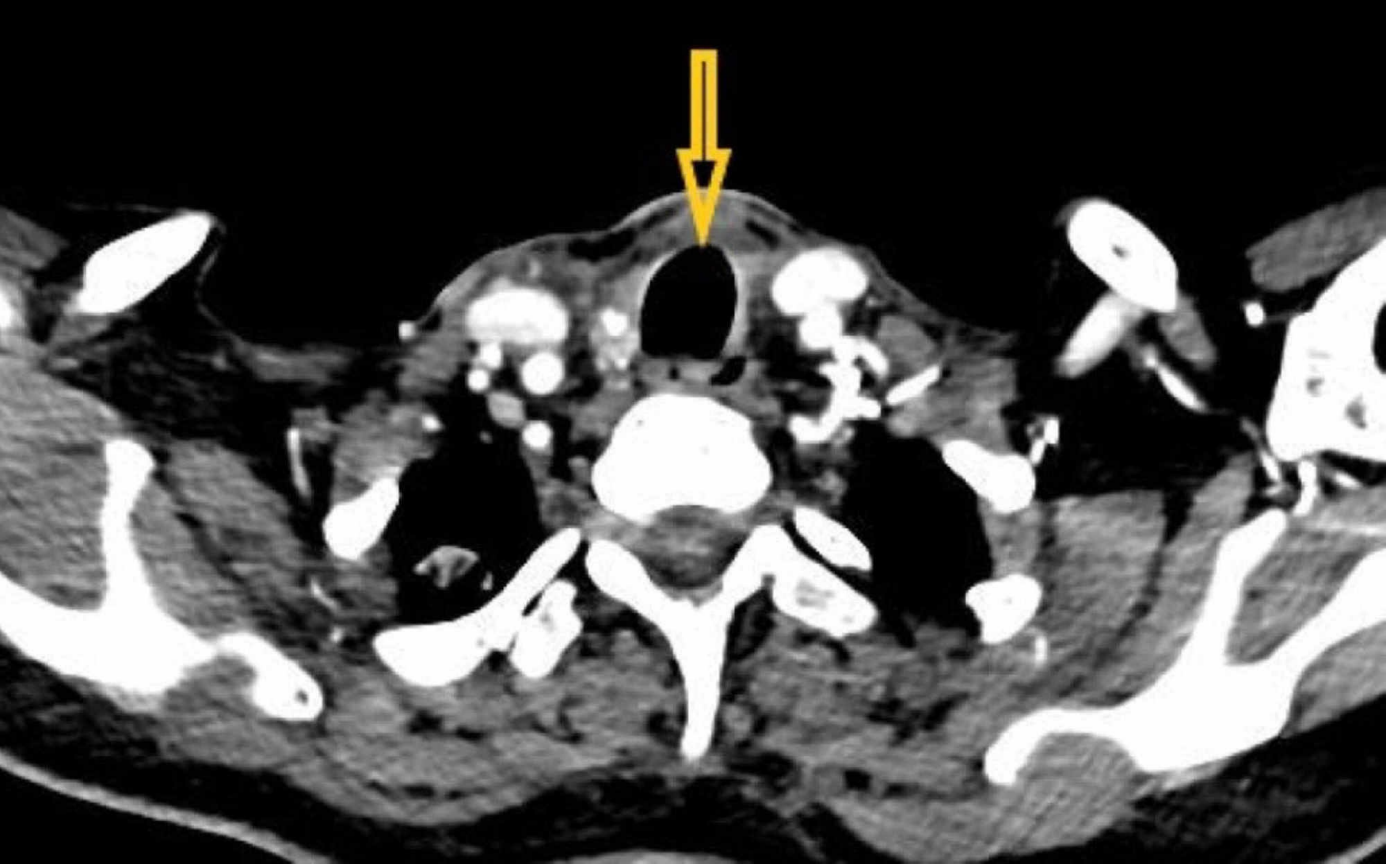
Postoperative axial CT scan section showing clearance of the disease and relief of tracheal compression (shown with the arrow).
